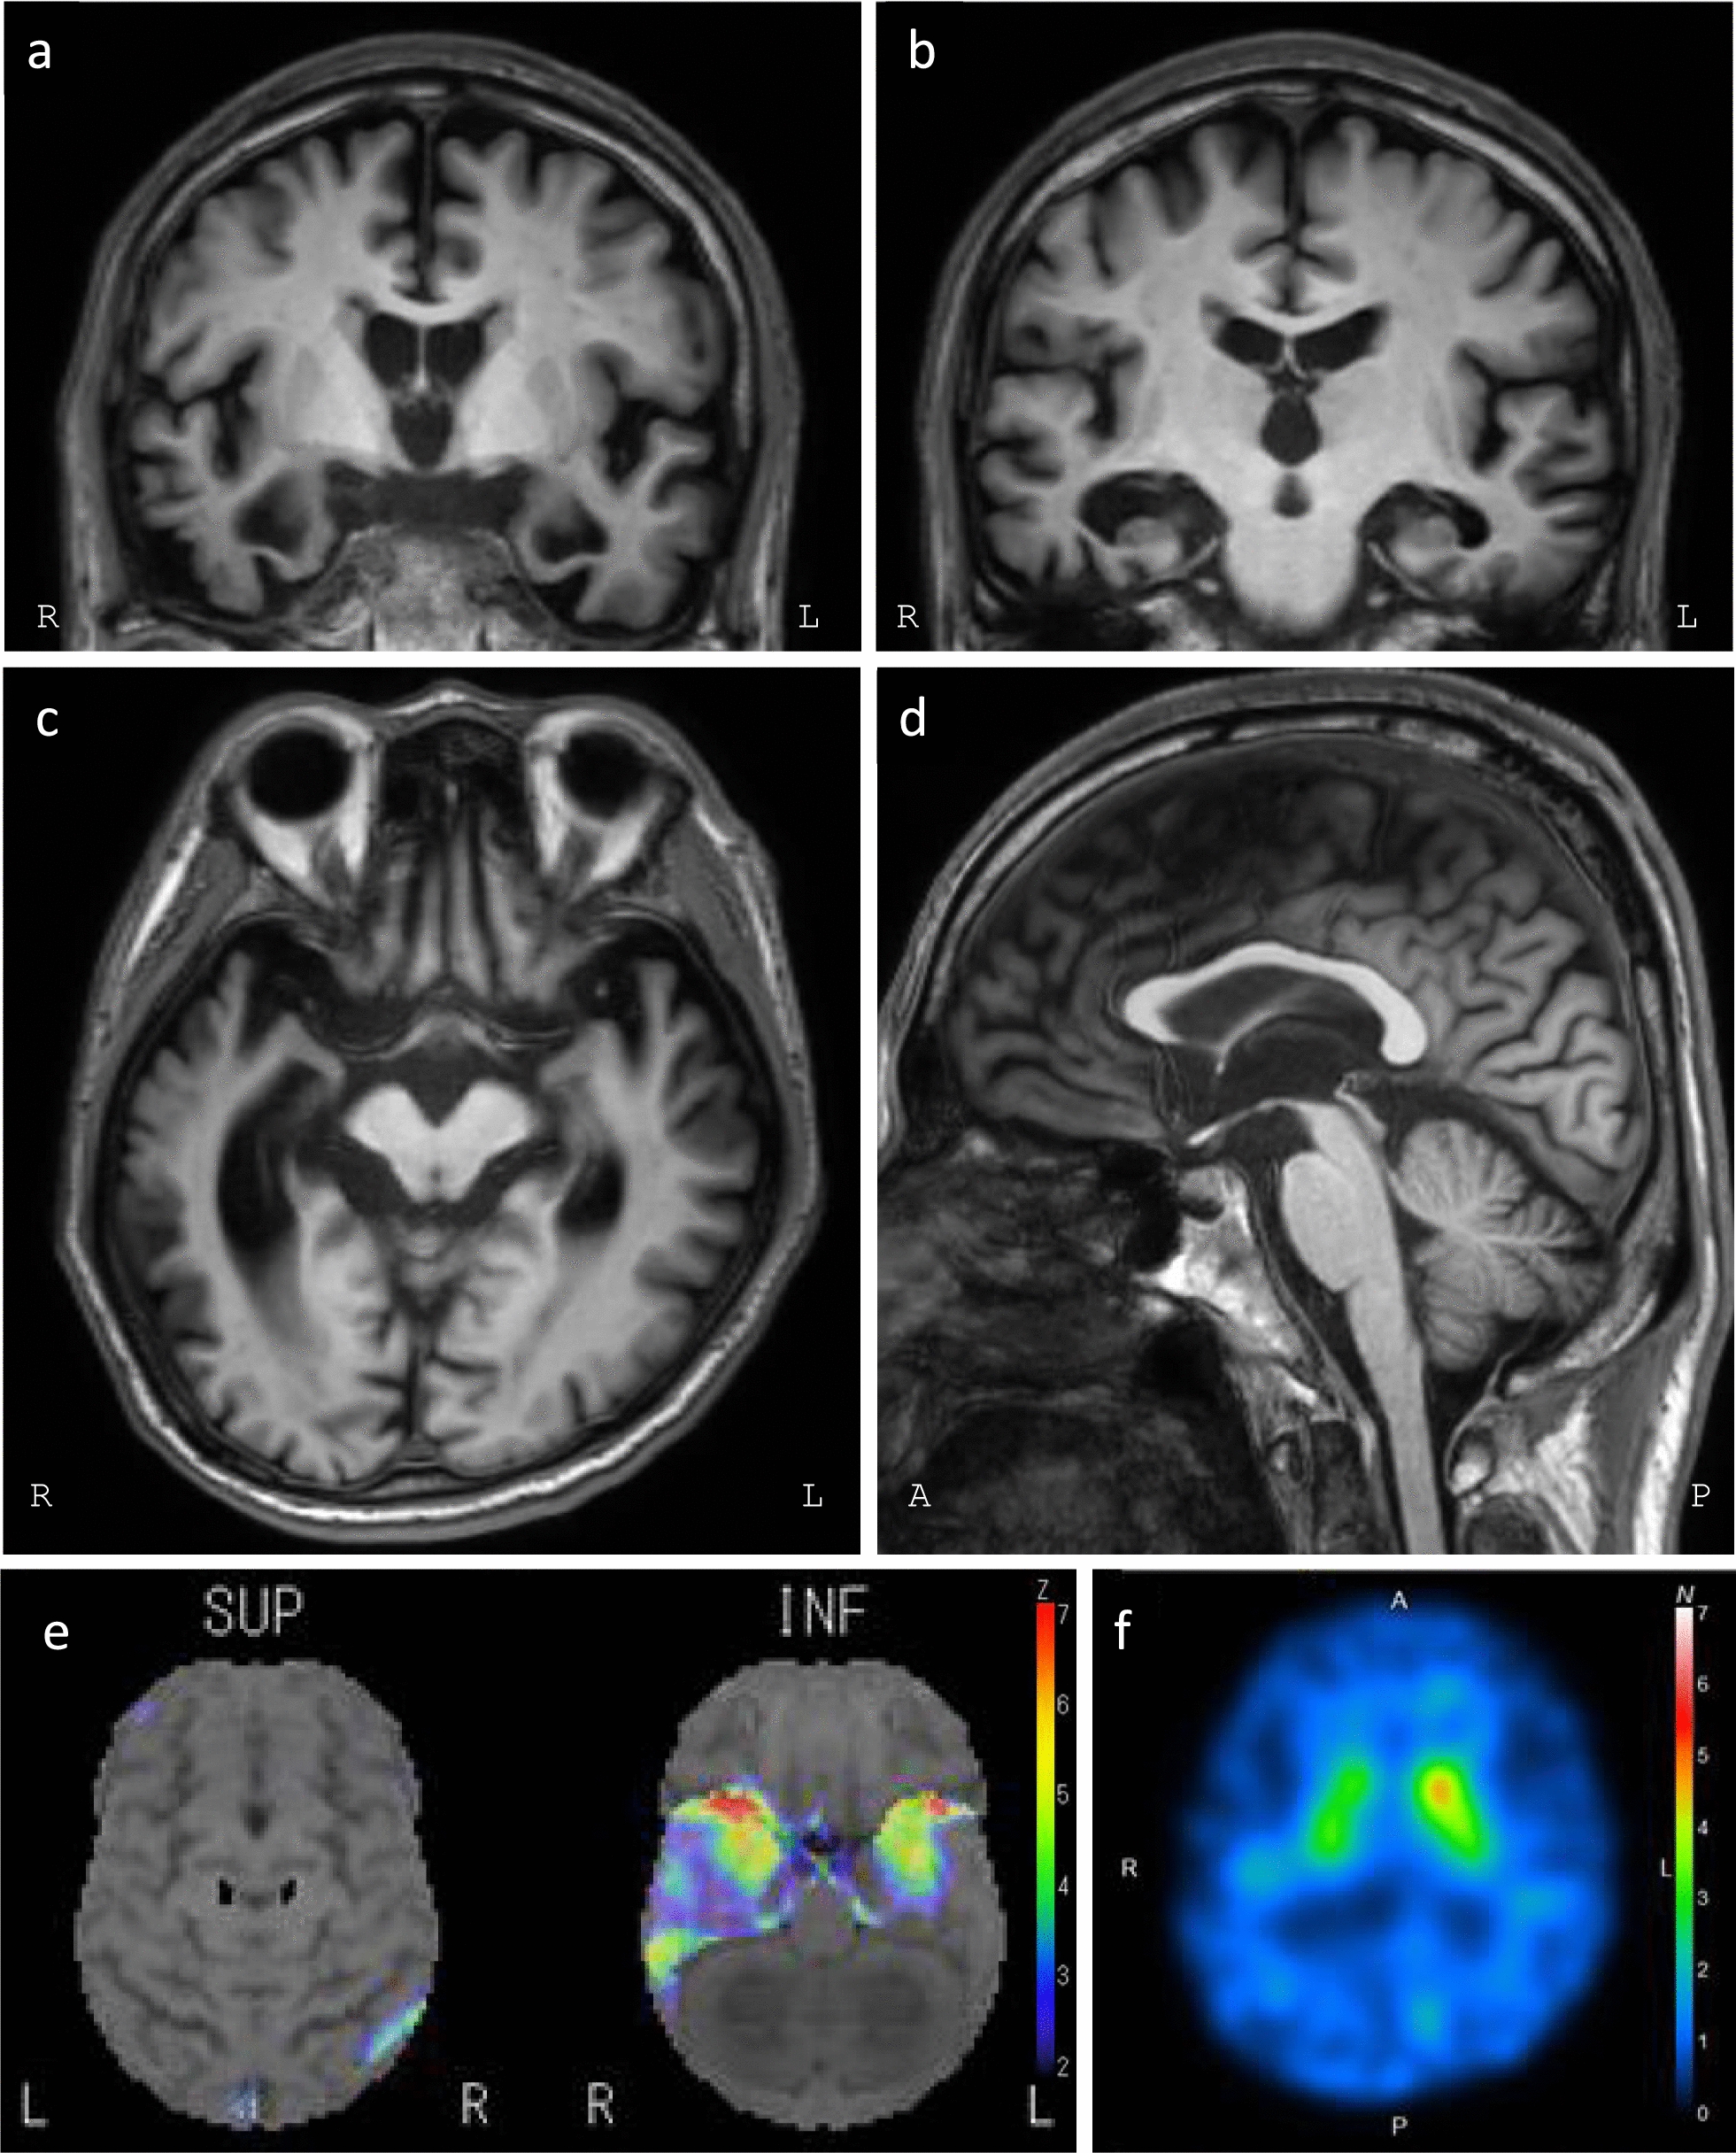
Clinical Images. a–d T1-weighted MR images at age 59. a Coronal image showing visible amygdala. Severe bilateral amygdala atrophy, predominantly on the right side, can be observed. White matter signal intensities in the left temporal lobe and right superior temporal gyrus are preserved, while those intensities in the right middle and inferior temporal gyri are attenuated. The third ventricle is enlarged. b Coronal image showing the hippocampal body. The hippocampus is mildly atrophic but relatively spared. c Axial image displaying predominantly enlarged right inferior horn of the lateral ventricle. Mild midbrain atrophy is noticeable. d Sagittal image revealing mild atrophy in the midbrain tegmentum area. e 3D-SSP image of ^123^I-IMP-SPECT demonstrates predominant right medial temporal hypoperfusion at age 57. f 123I-Ioflupane-SPECT reveals reduced binding to the striatal dopamine transporter, predominantly on the right side, at age 57
